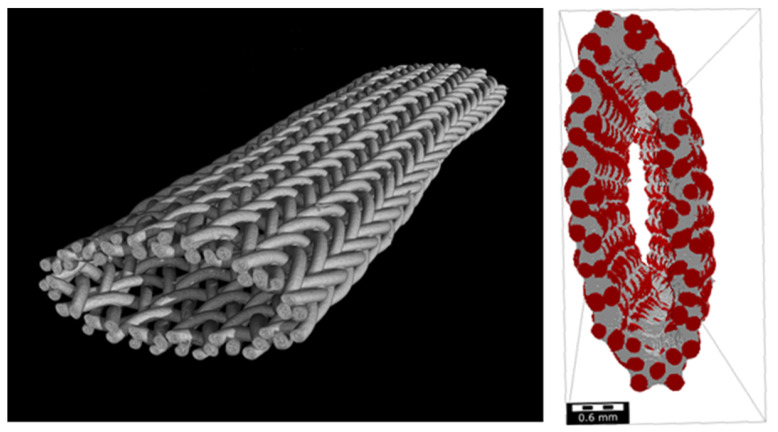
Example of a µ-CT scan (from a braided scaffold consisting of round monofilaments) including the area fraction of pores (gray) and fibers (red).
